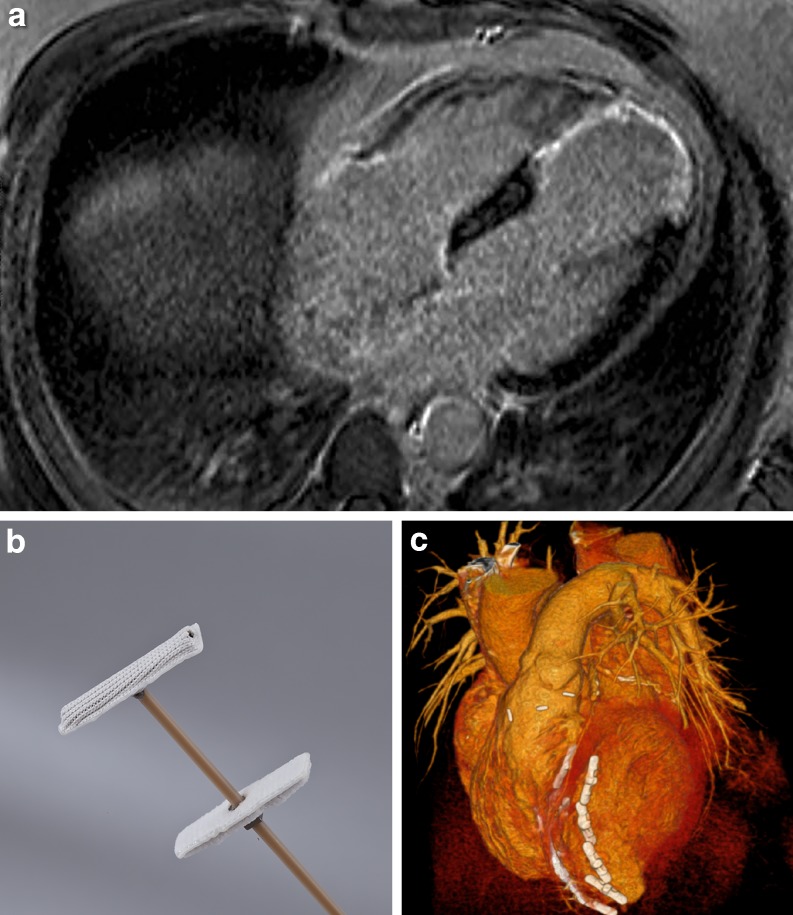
Left ventricular remodelling. a Transversal plane of magnetic resonance imaging showing left ventricular aneurysm caused by apical myocardial infarction, as visualised by late enhancement. b,c Revivent TC Ventricular Enhancement System (BioVentrix; San Ramon, CA, USA). Internal and external anchors of the device work together to exclude scarred tissue of the left ventricle (b). A computed tomography scan reconstruction of the heart (anterior view) after the implantation of a series of anchor pairs (c). (Images adapted with permission from BioVentrix; San Ramon, CA, USA)
